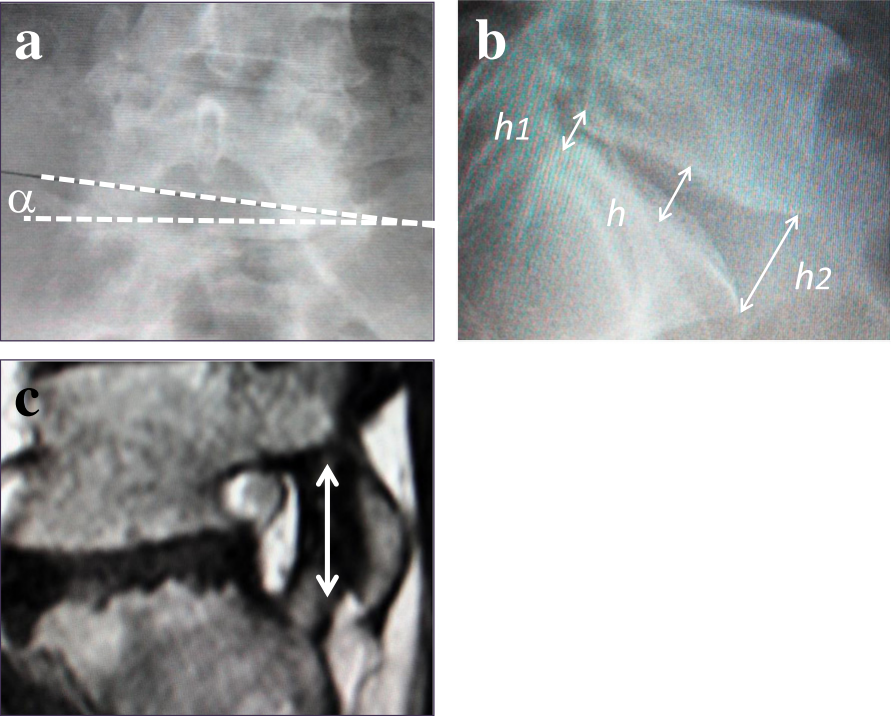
Radiographic parameters. a L5-S1 wedging angle in a frontal standing plain X-ray. b Disc height of L5-S1 intervertebral disc: *h* = (*h1* + *h2*) × 1/2. c Foraminal ratio: bilateral L5-S1 foraminal ratio of the narrower to the wider foraminal craniocaudal diameter
